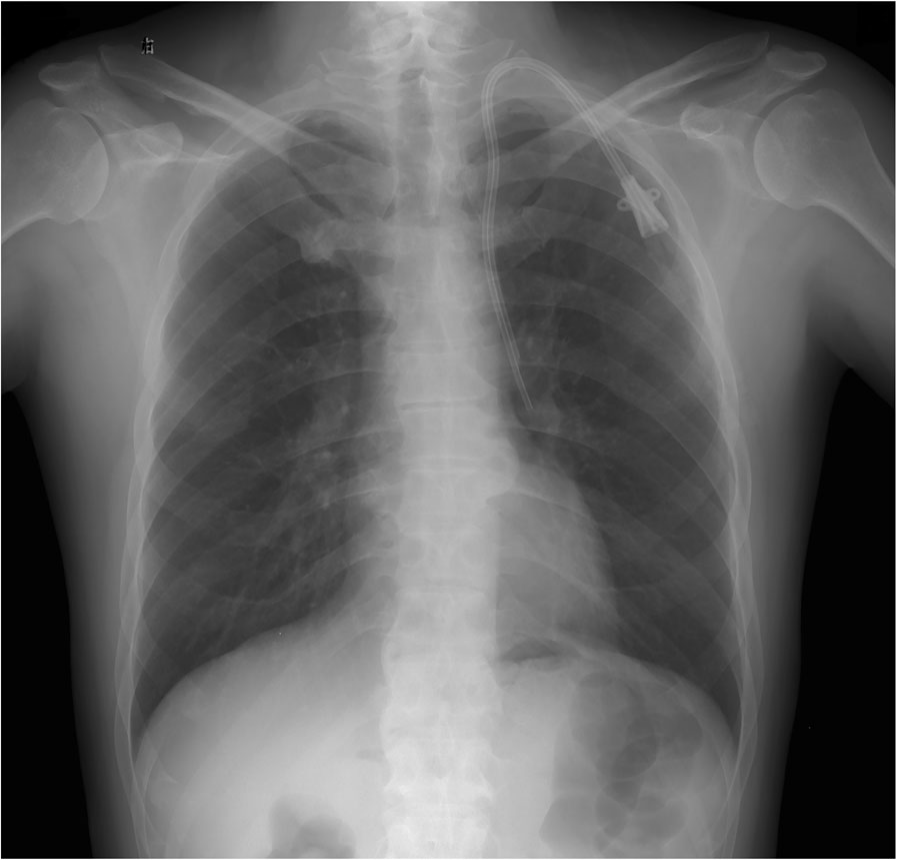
Postoperative chest radiograph showed the location of the cuffed, tunneled hemodialysis catheter and its abnormal path
